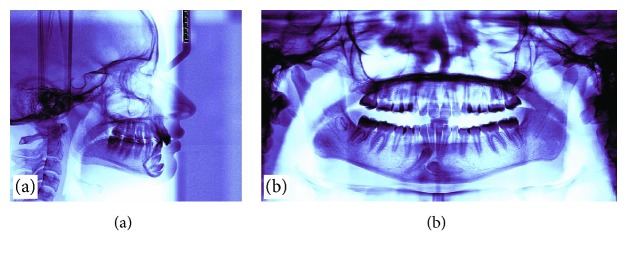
(a) Pretreatment lateral cephalogram. (b) Pretreatment panoramic radiograph.
